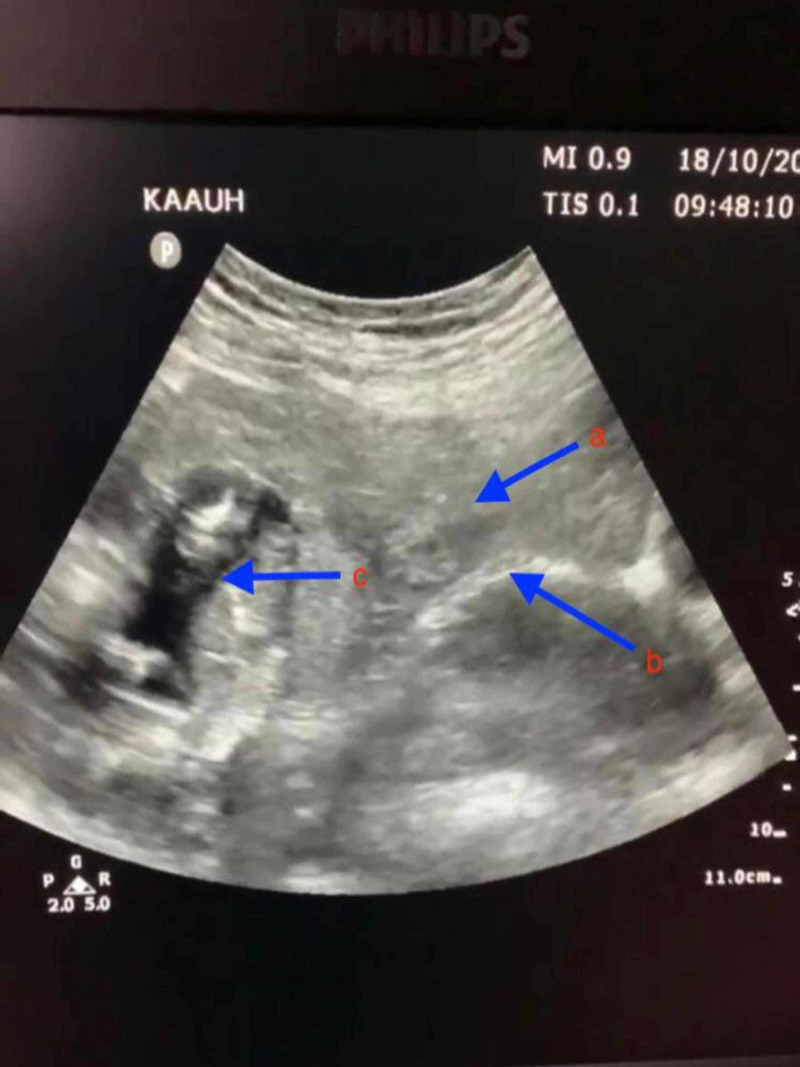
Trans-abdominal ultrasound of pelvis Arrows indicate: (a) uterus; (b) endometrial line; (c) fetus.
